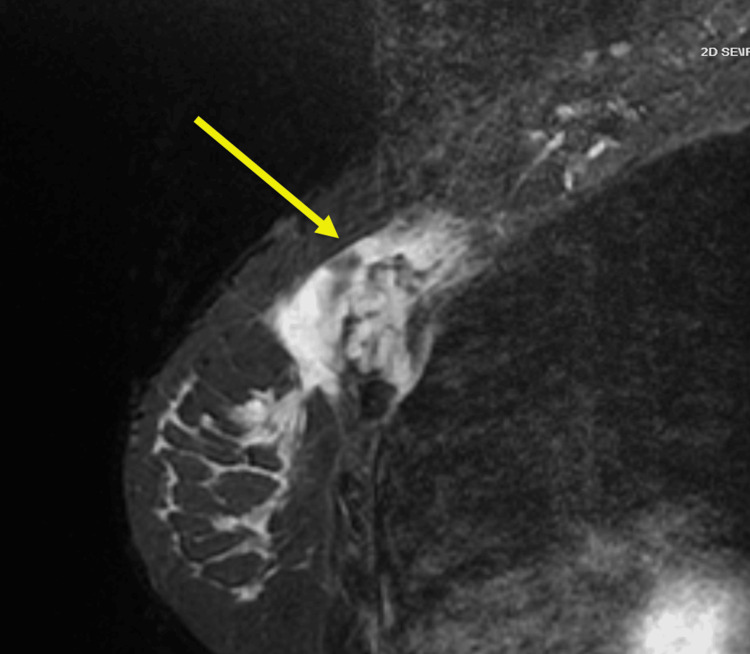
CT Thorax with contrast in the sagittal plane which demonstrates a partially enhancing spiculated mass in the left anterior chest wall with associated skin thickening and irregularity.
